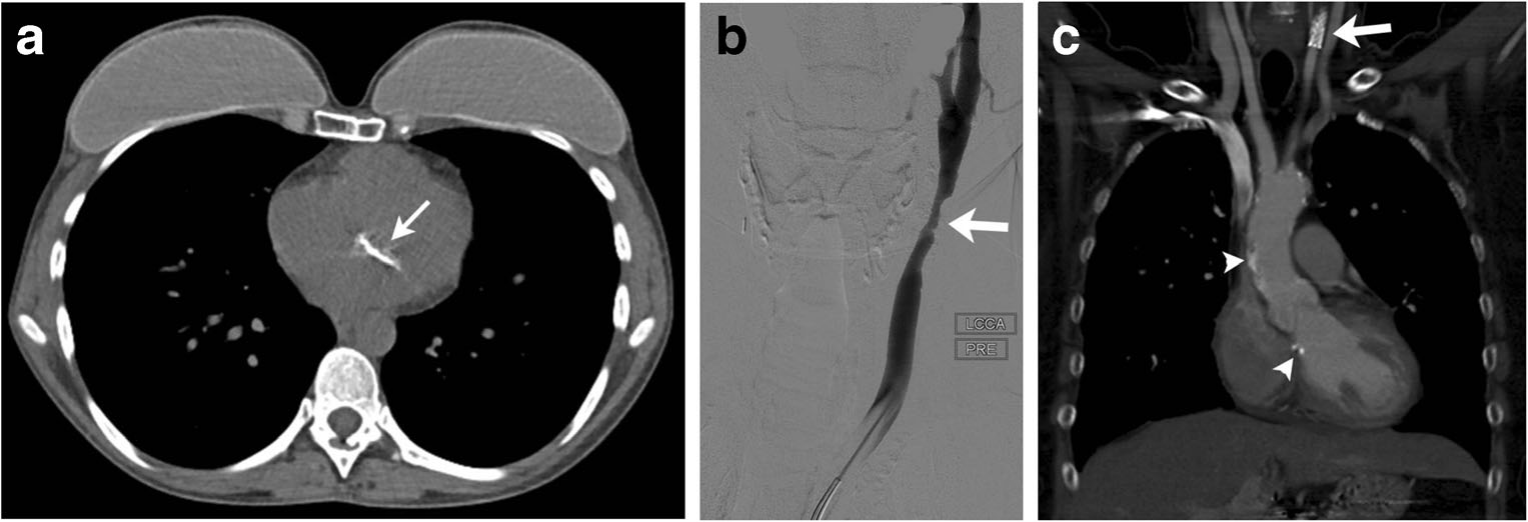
Cardiovascular complications. a A 38-year-old female with a history of nodular sclerosing HL at the age of 12 treated with chemotherapy and upper mantle radiation. Axial non-enhanced CT image shows mitral valve calcification (*arrow*). Note the bilateral mastectomies with a breast prosthesis from prior breast cancer. b A 40-year-old female with a history of nodular sclerosing HL at the age of 15 treated with chemotherapy and upper mantle radiation. Digital subtraction angiogram of the left carotid artery shows moderate to severe narrowing of the left distal common carotid artery (*arrow*). c Coronal reformatted image of contrast-enhanced CT during follow-up shows the presence of a stent in the left common carotid artery (*arrow*). Note the presence of aortic arch and right coronary artery calcifications (*arrowheads*). Also note the absence of spleen from the prior splenectomy
